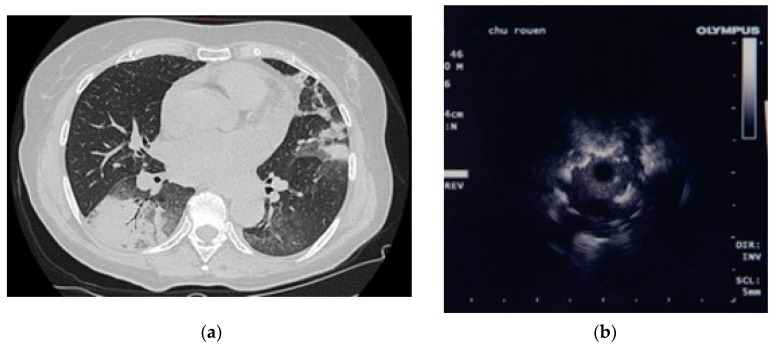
CT-scan image of organizing pneumonia with bilateral alveolar opacities (a). Ultrasonographic image of organizing pneumonia (b).
